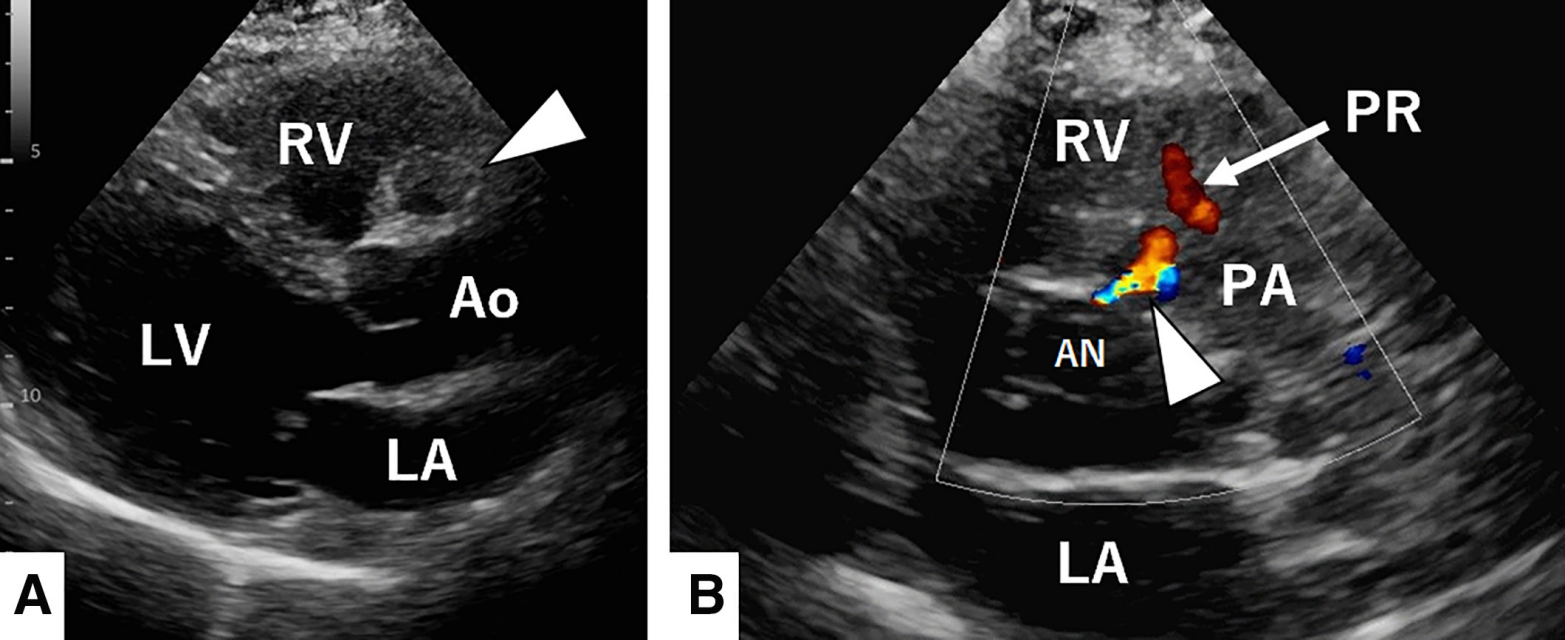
Transthoracic echocardiographic findings. Transthoracic echocardiography reveals a round structure measuring 19 × 13 mm near the right sinus of Valsalva (arrowhead) (A: long-axis view) and also demonstrates diastolic flow entering the main pulmonary artery immediately above the pulmonary valve (arrowhead) (B: short-axis view). AN, aneurysm; Ao, aorta; LA, left atrium; LV, left ventricle; PA, pulmonary artery; PR, pulmonary valve regurgitation; RV, right ventricle
